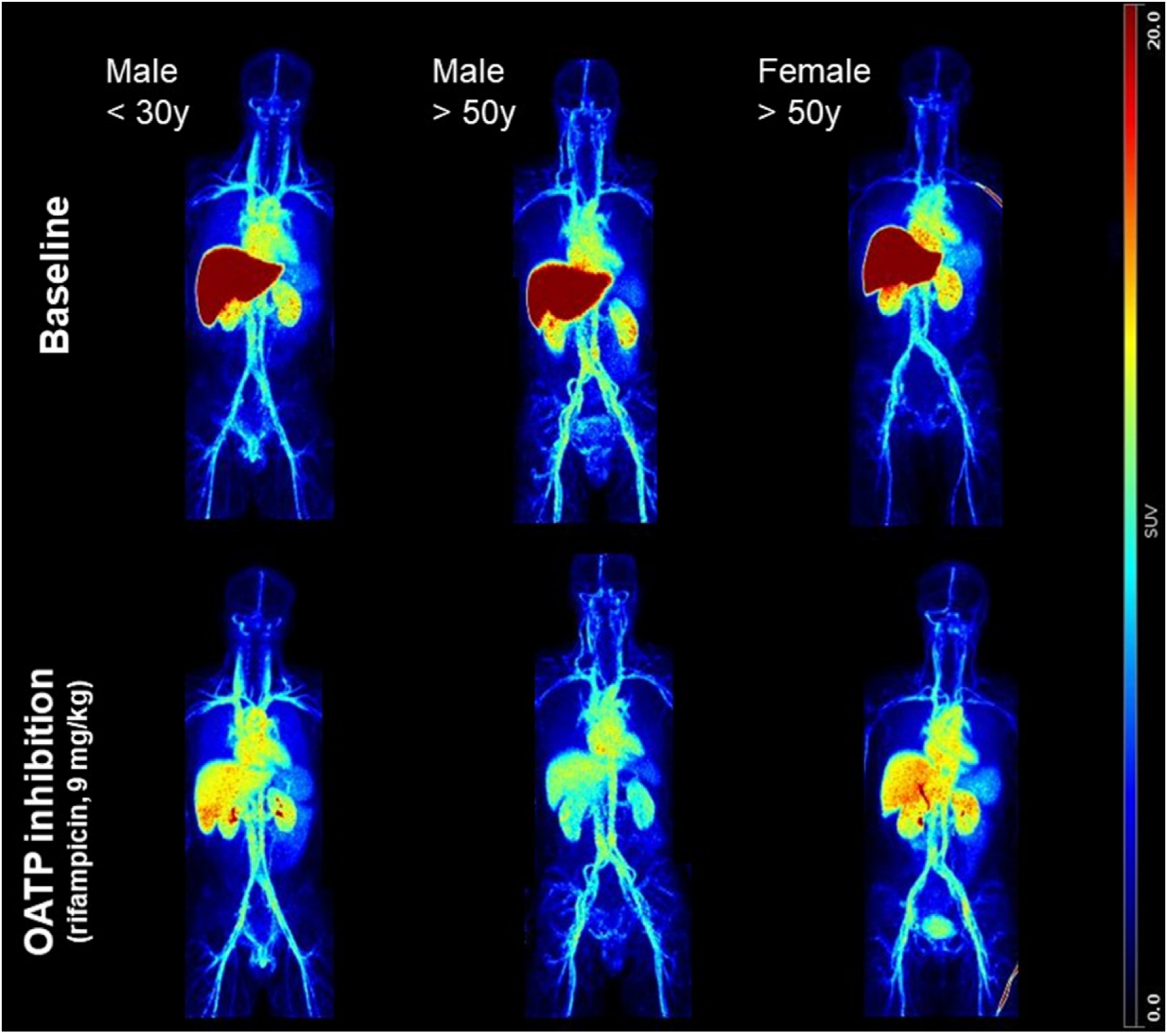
Whole-body PET images of ^11^C-glyburide distribution in baseline condition and after treatment with rifampicin (9 mg/kg i.v.) according to age and sex. Images are the maximum intensity projections of the frames summed from 0 to 30 min and are expressed in standardized uptake values (SUV).
